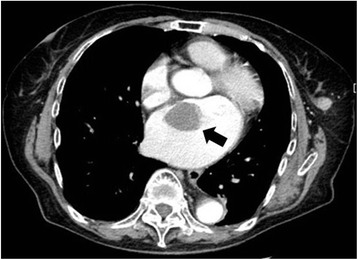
A 30 × 30-mm mass in the left atrium on contrast-enhanced computed tomography of the chest (black arrow)
